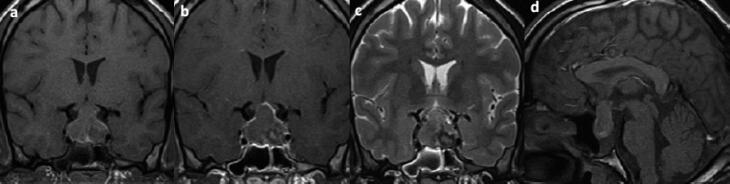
MRI at 22 years of age in coronal T1W (a), post-contrast T1W (b), coronal T2W (c) and sagittal T1W (d) views showing a 3.3 × 2.4 × 1.4 cm sellar lesion, with suprasellar extension and optic chiasm compression, heterogeneous, predominantly isointense in T1W and T2W, with peripheral contrast enhancement, exhibiting an internal small area with hypointense rim in T2W, suggestive of pituitary adenoma with hemorrhagic degeneration in different stages of evolution.
